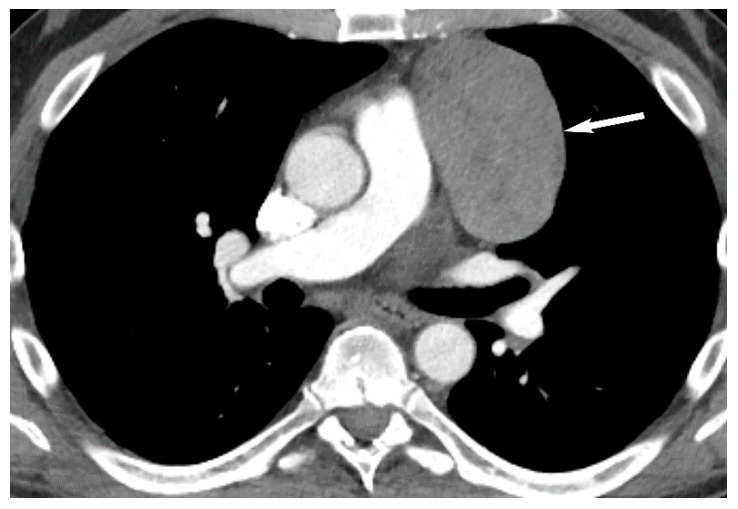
Thymoma. CT shows a well-defined homogeneous soft tissue mass in the prevascular mediastinum (arrow).
